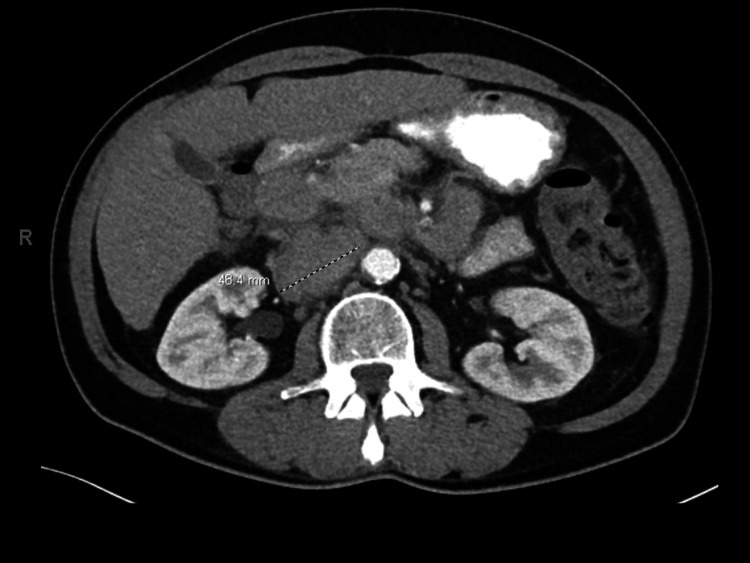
CT scan done in previous workup revealing 5cm large soft tissue attenuation suspicious of malignancy.
